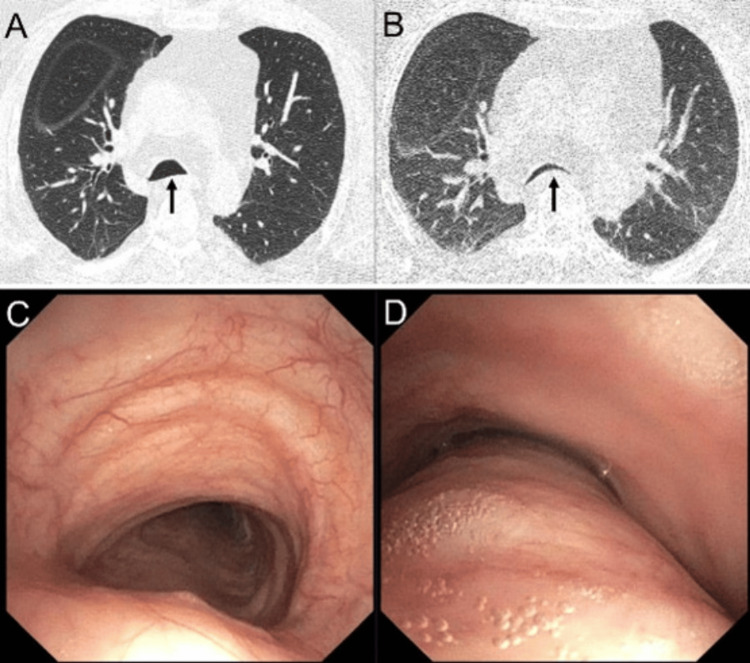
Tracheomalacia. Axial CT images during inspiration (A) and expiration (B) show a flattened tracheal morphology during both phases, with more than 70% reduction in anterior-posterior diameter during expiration (arrows) and patchy expiratory air trapping. Bronchoscopy images during inspiration (C) and expiration (D) reveal tracheal collapse during expiration.
